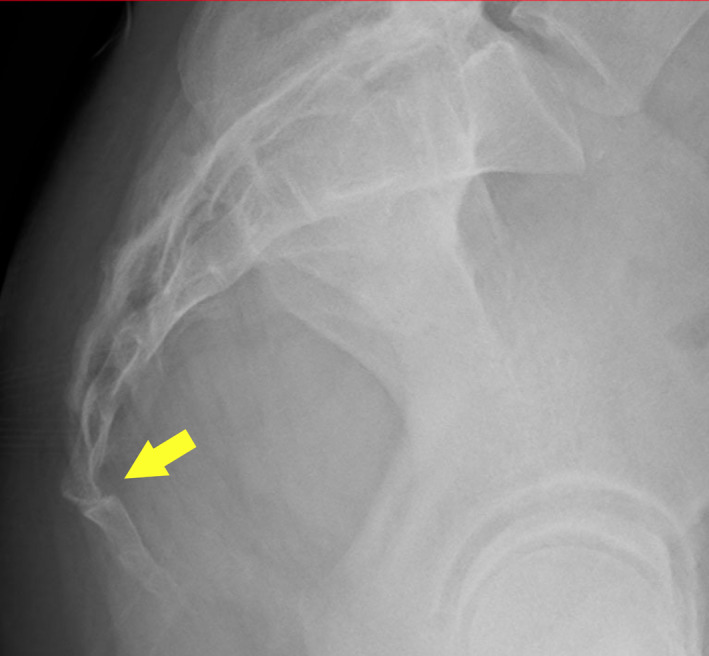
Roentgenogram of the sacrococcygeal vertebrae showing subluxation of the coccyx to the ventral side (*arrow*)
